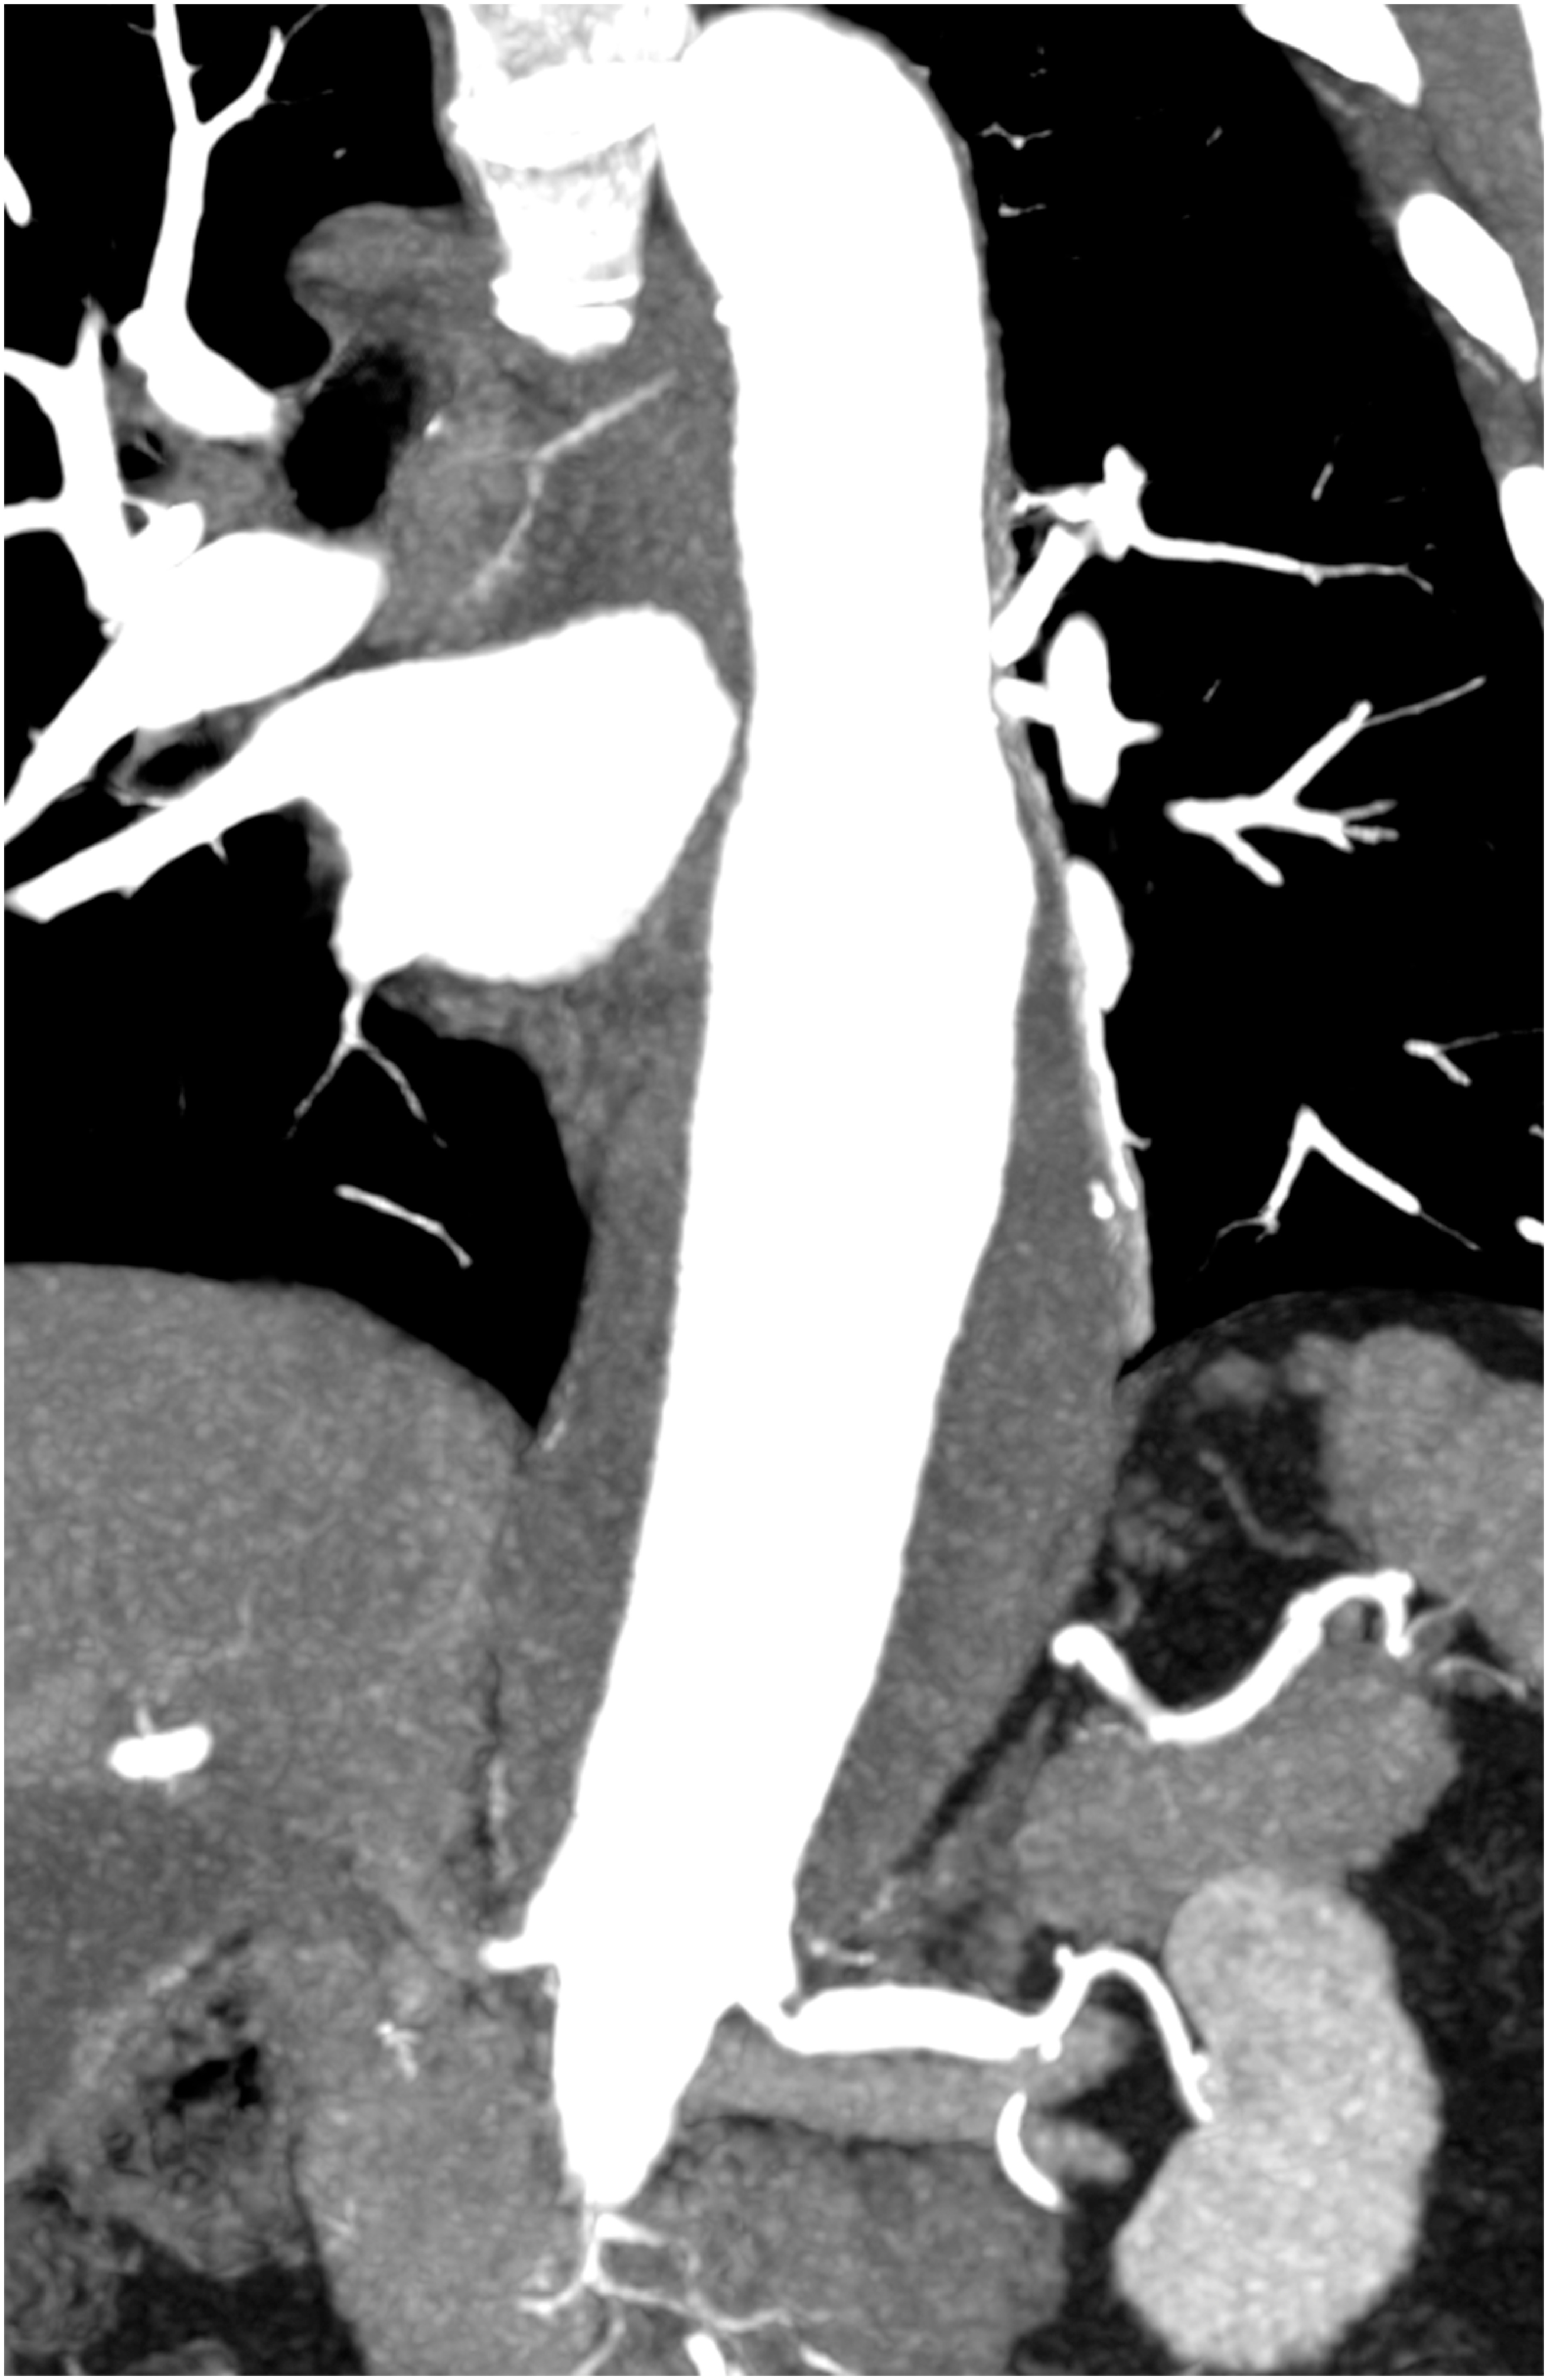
Fig. 1 Pre-operative computed tomography angiogram.
